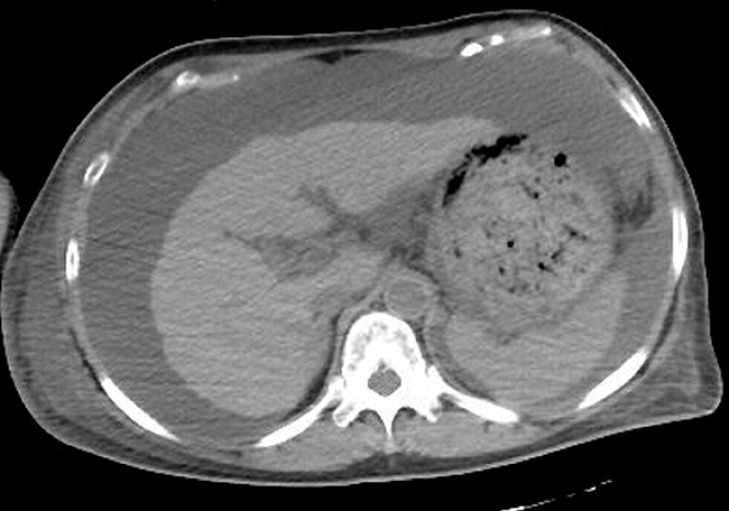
Plain abdominal computed tomography scan showing liver cirrhosis with massive ascites.
